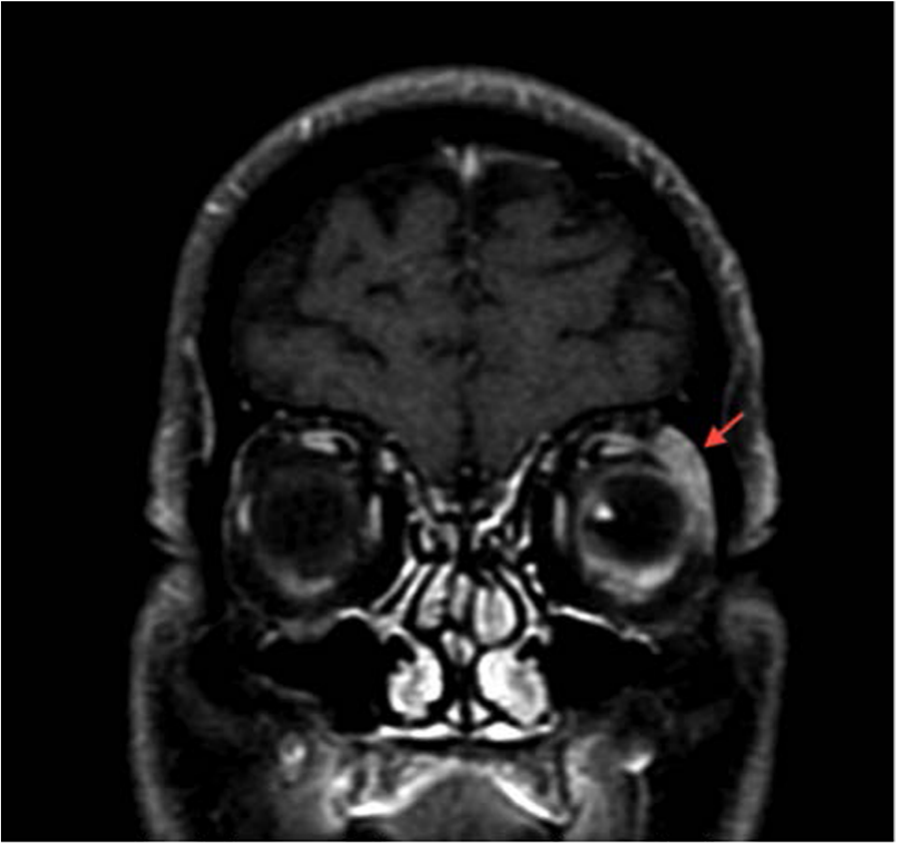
Coronal T1-weighted magnetic resonance imaging scan. Contrast agent was injected with fat suppression mode on. Peri-bulbar inflammation is more evident at the left lacrimal gland (red arrow).
